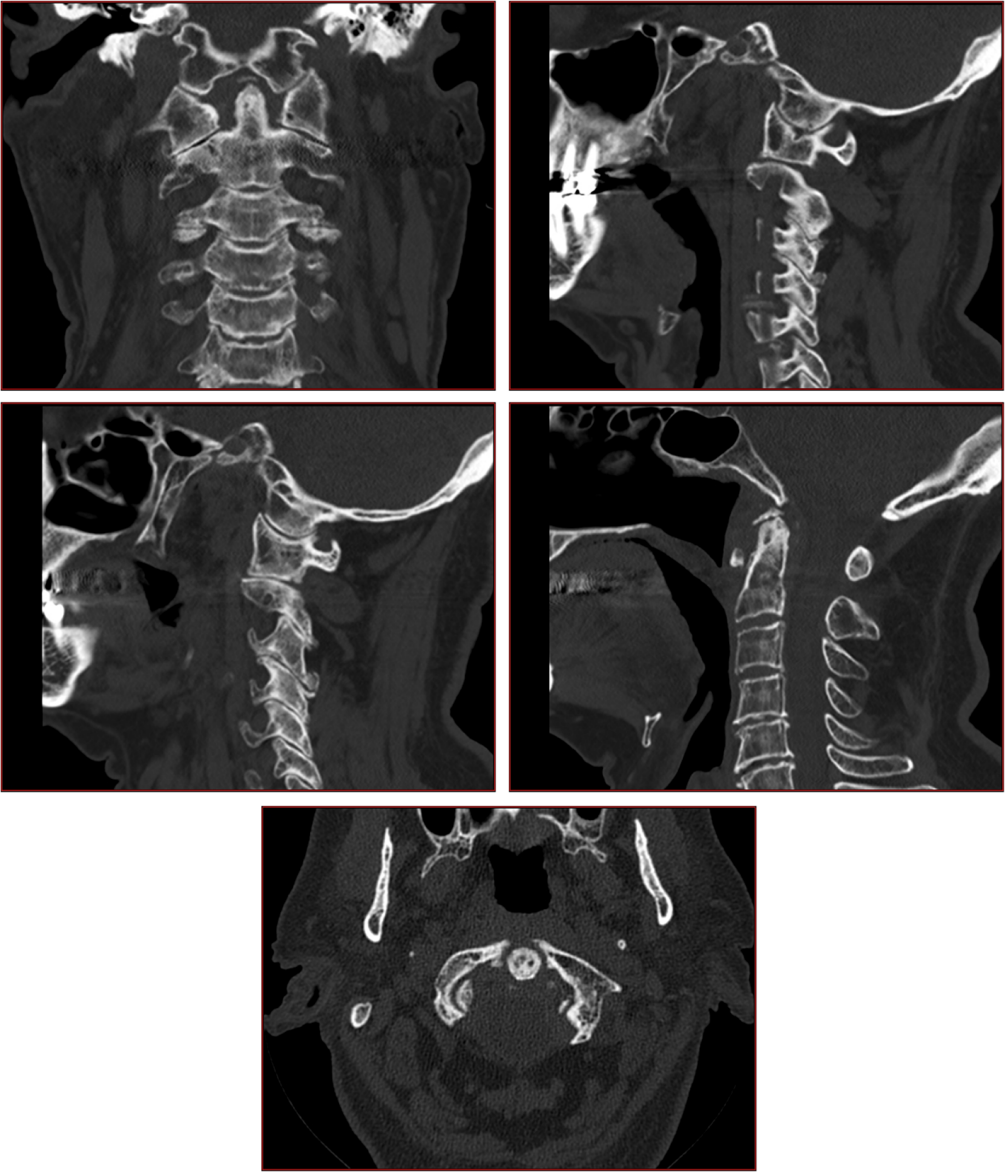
8-month follow-up CT slices demonstrating a maintained reduction of the anterior arch of C1 and subtle signs of healing on the left side of the fracture of the anterior arch of C1. a. Coronal slice through the dens of C2. b. Right-sided parasagittal slice through the C1-C2 joint. c. Left-sided parasagittal slice through the C1-C2 joint. d. Mid-sagittal slice displaying a normalized ADI. e). Axial slice through the tip of C2
